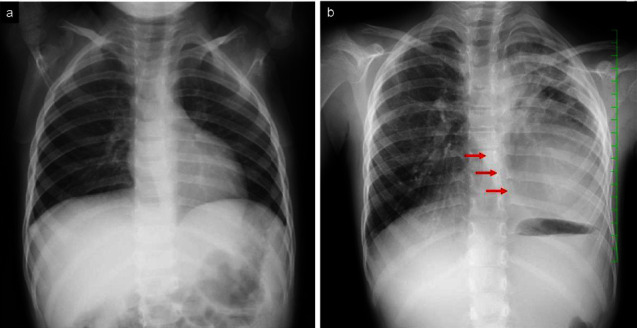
(a) Chest radiography at 2 years of age (at the time of discharge). (b) Mediastinal shift to the left side due to the volume loss of left lung (red arrows) at the age of 9 years.
